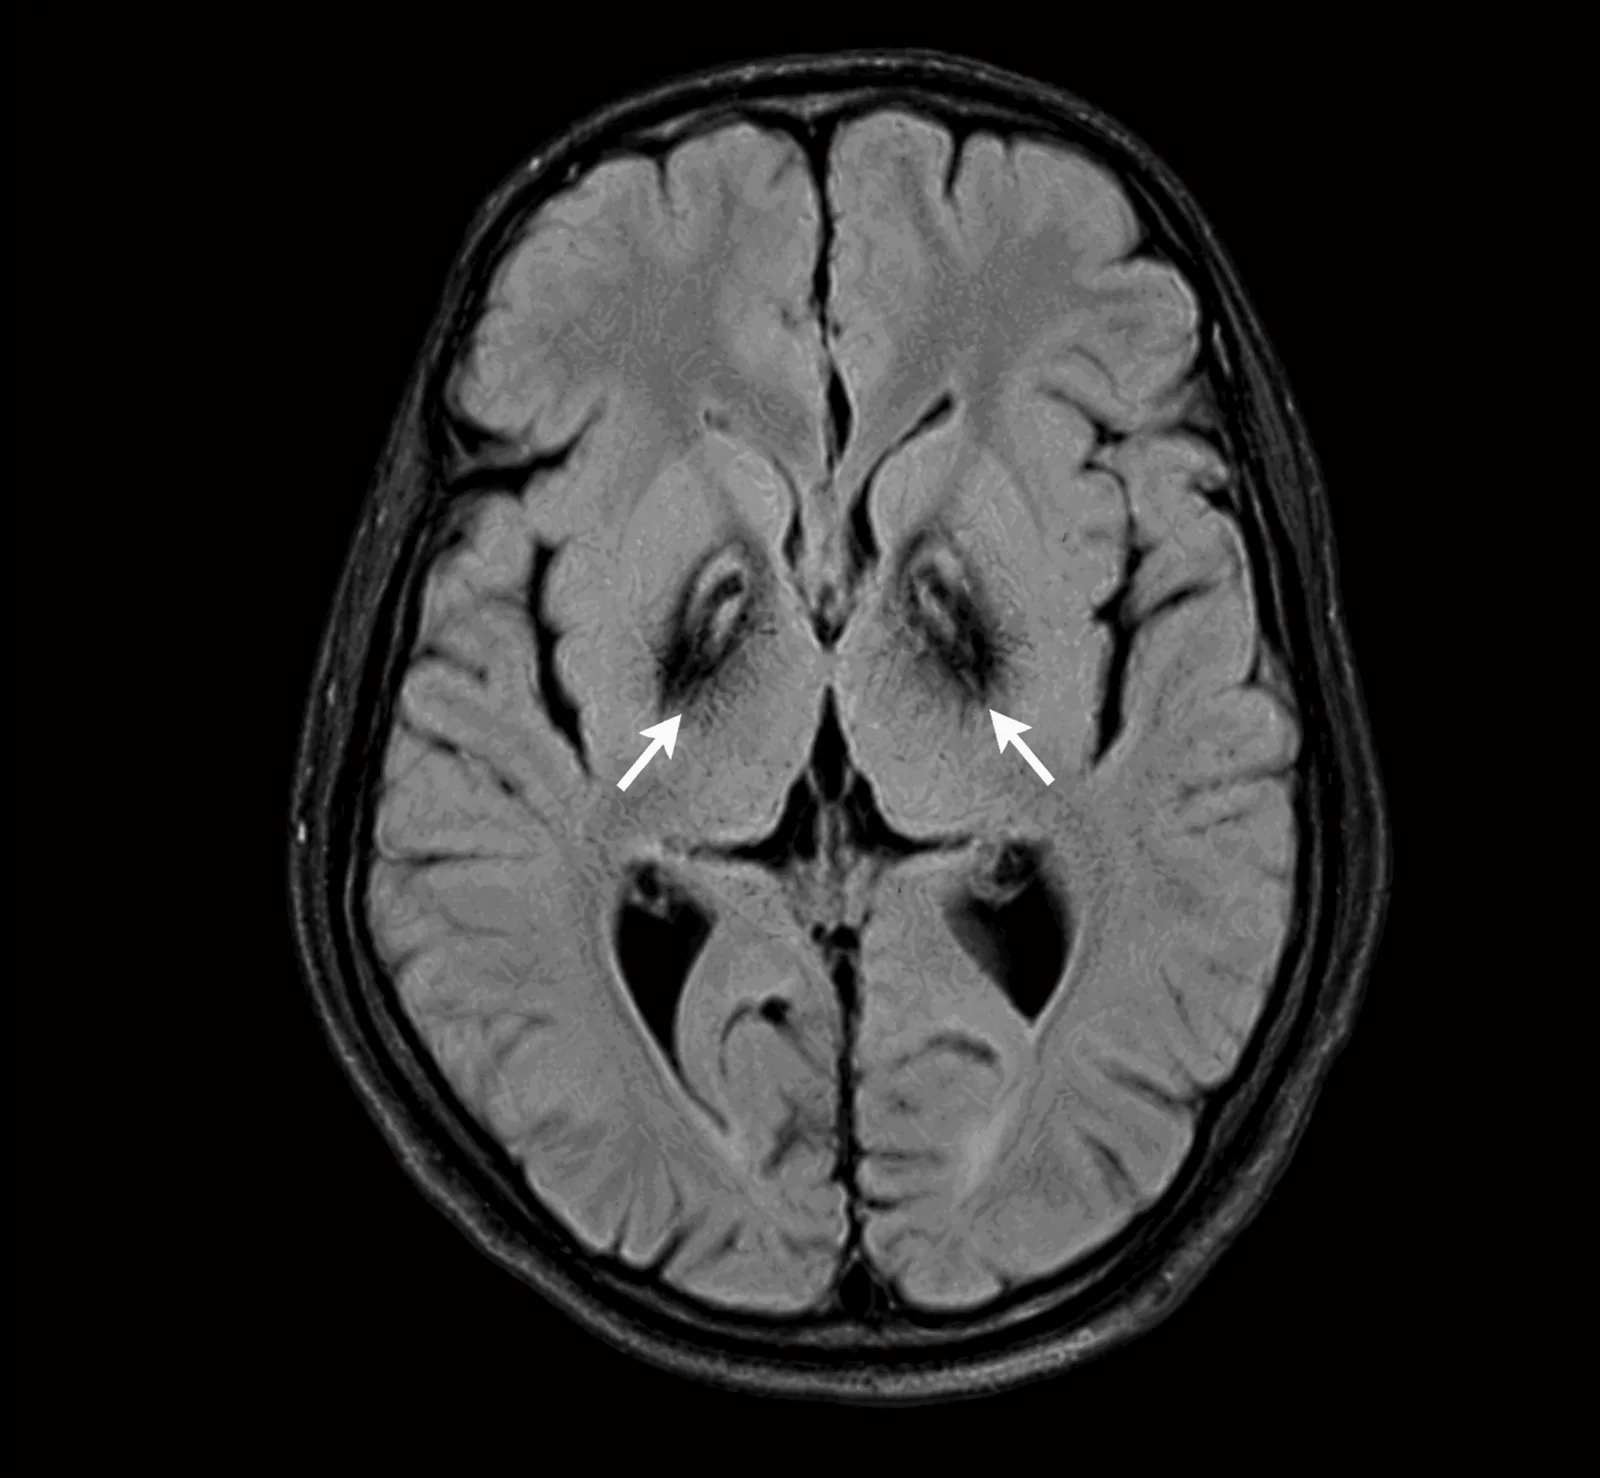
Axial susceptibility-weighted imaging (SWI) demonstrating prominent blooming artifacts in the bilateral globus pallidus (white arrows), consistent with iron accumulation. These findings support basal ganglia iron deposition and highlight the utility of SWI in detecting pathological changes in PKAN, particularly in the absence of classic T2-weighted MRI findings. PKAN, pantothenate kinase-associated neurodegeneration
